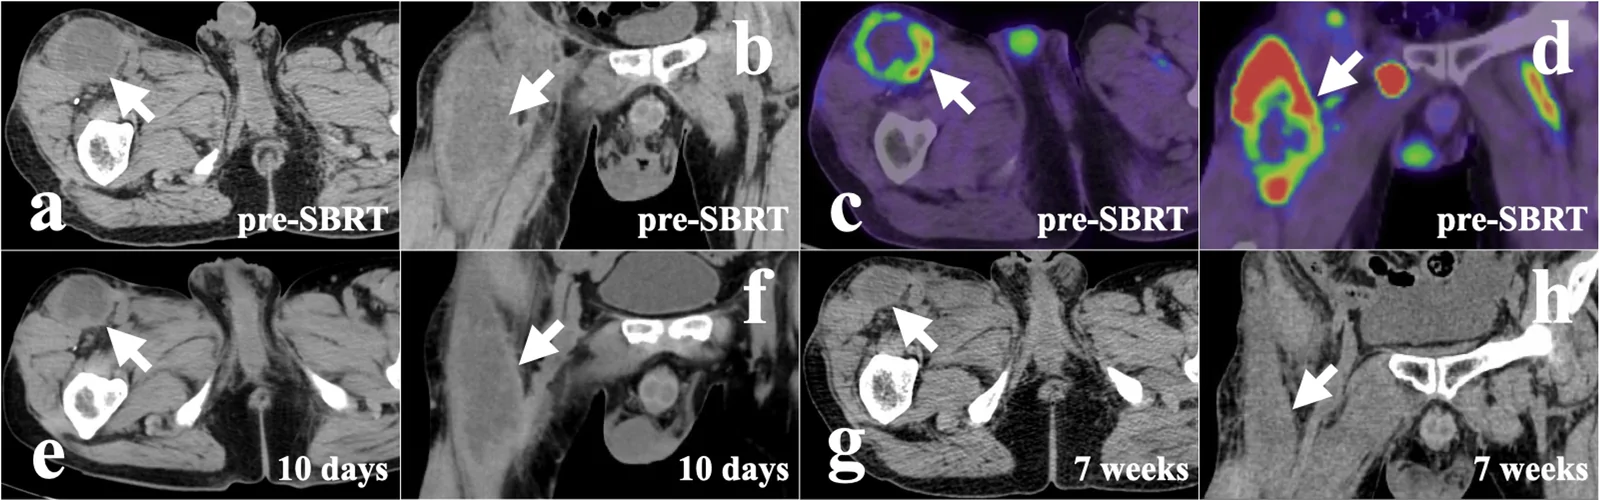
Metastatic lesion in the right thigh (white arrows). (a-d) Images obtained before SBRT. (a, b) Non-contrast CT showing the lesion with an isodense wall and internal low density. (c, d) PET/CT demonstrating intense FDG uptake in the wall of the lesion. (e, f) Non-contrast CT obtained 10 days after SBRT initiation (the final day of treatment), showing tumor regression. (g, h) Non-contrast CT obtained seven weeks after SBRT, showing further tumor regression. (a, c, e, g) Axial views and (b, d, f, h) coronal views. SBRT, stereotactic body radiotherapy; CT, computed tomography; PET, positron emission tomography; FDG, 2-deoxy-2-[¹⁸F]fluoro-D-glucose.
